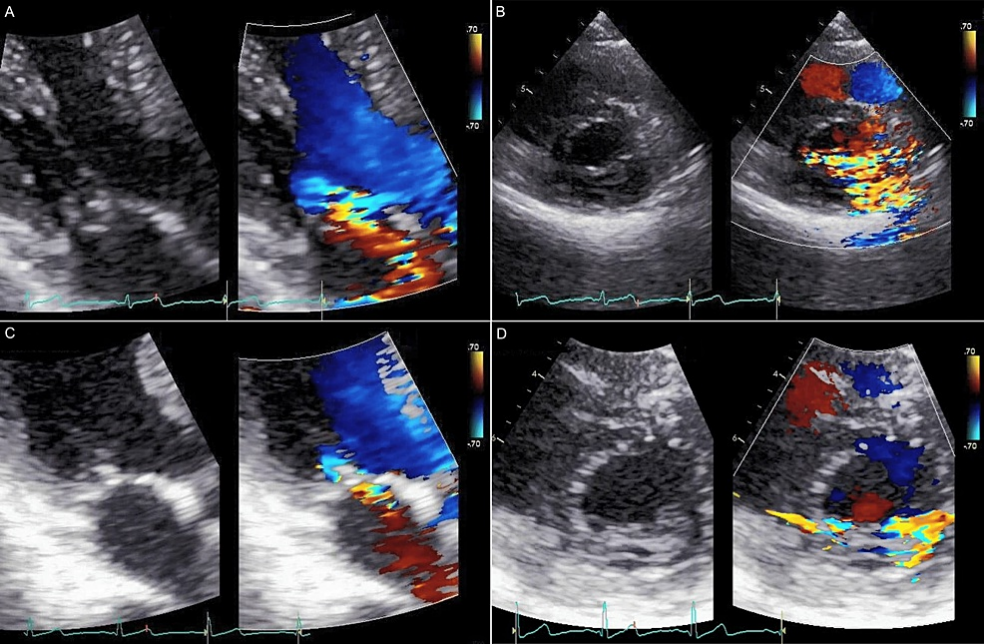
Echocardiography showing mitral regurgitation due to mitral valve prolapse at a presentation on apical three-chamber images (A) and short-axis images (B). The severity of mitral regurgitation improved three years after presentation (C, D).
